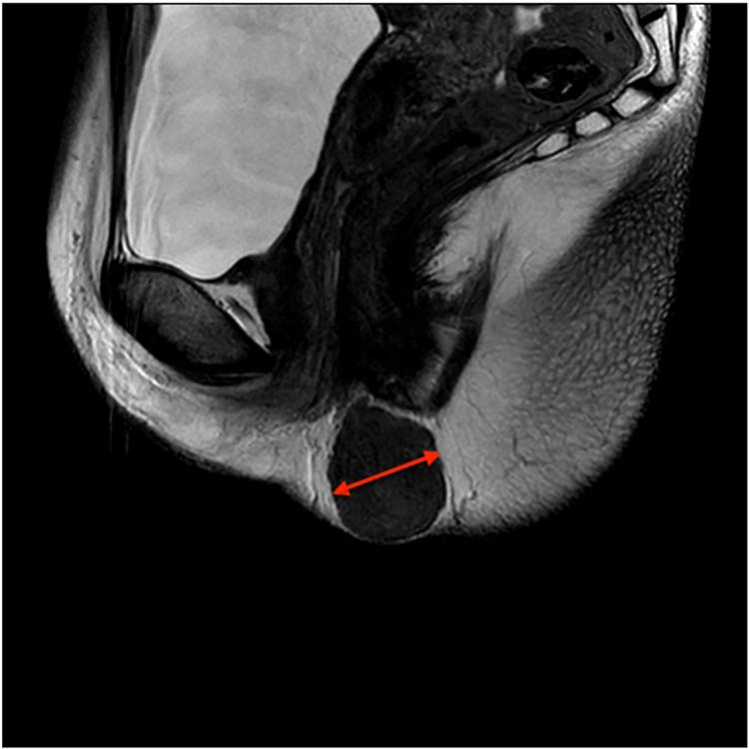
Coronal view MRI image of the lesion marked with a red arrow.
